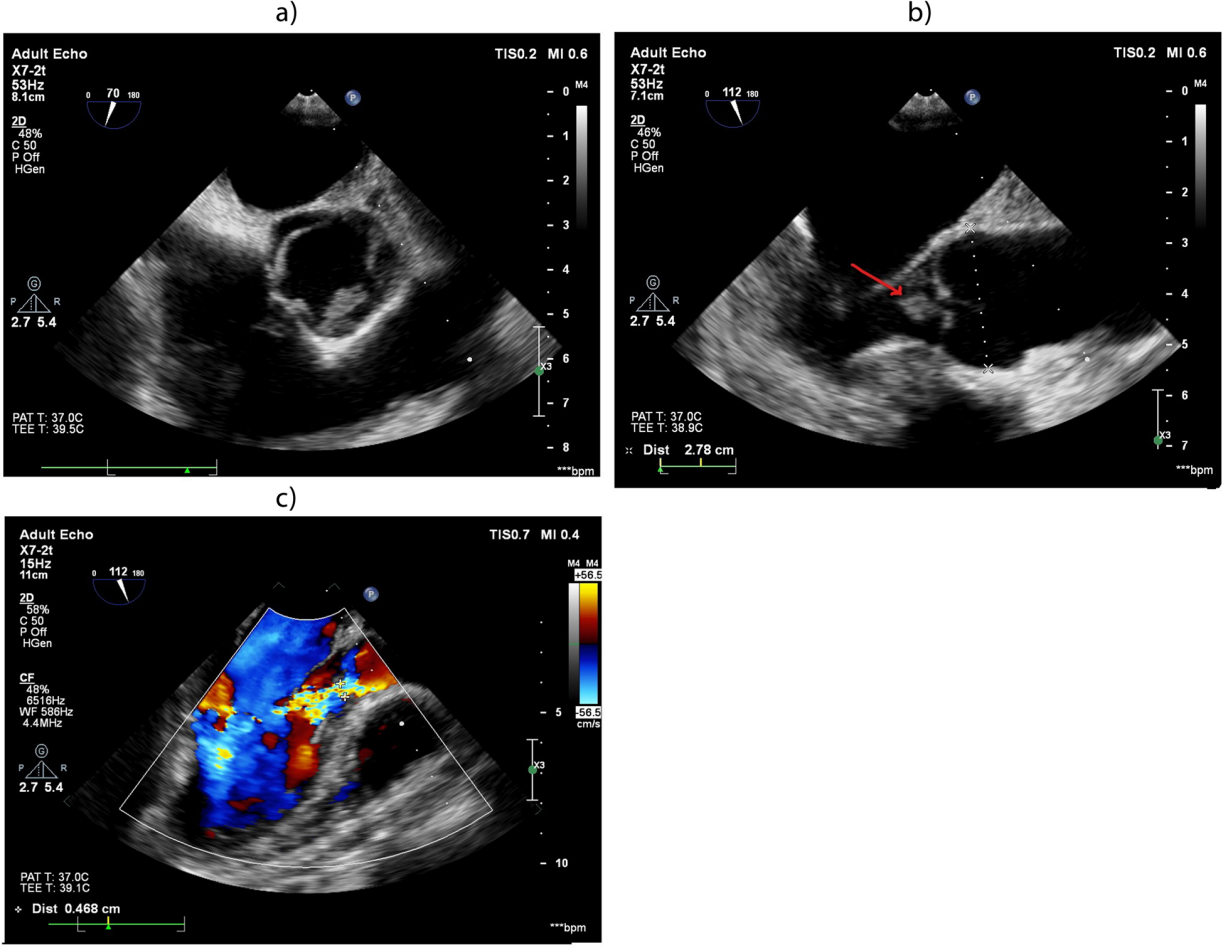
TOE, a Quadricuspid aortic valve, b Arrow, A vegetation attached to the aortic valve leaflet, c Doppler showing AR colour jet
